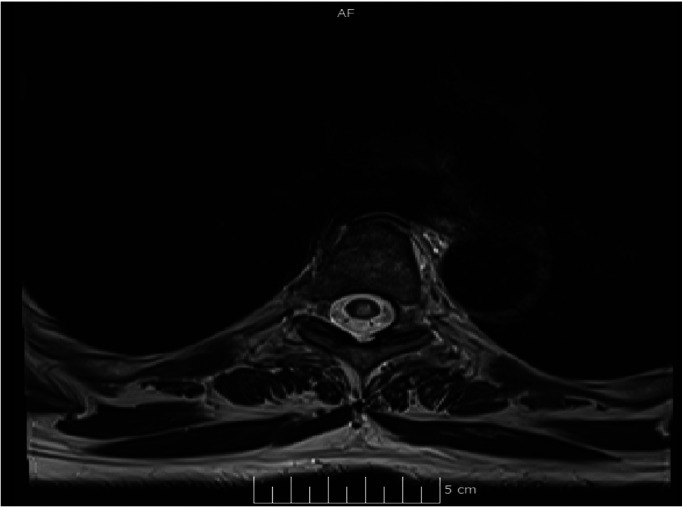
MRI of the thoracic spine in axial view with a non-enhancing intramedullary lesion suggestive of transverse myelitis.
